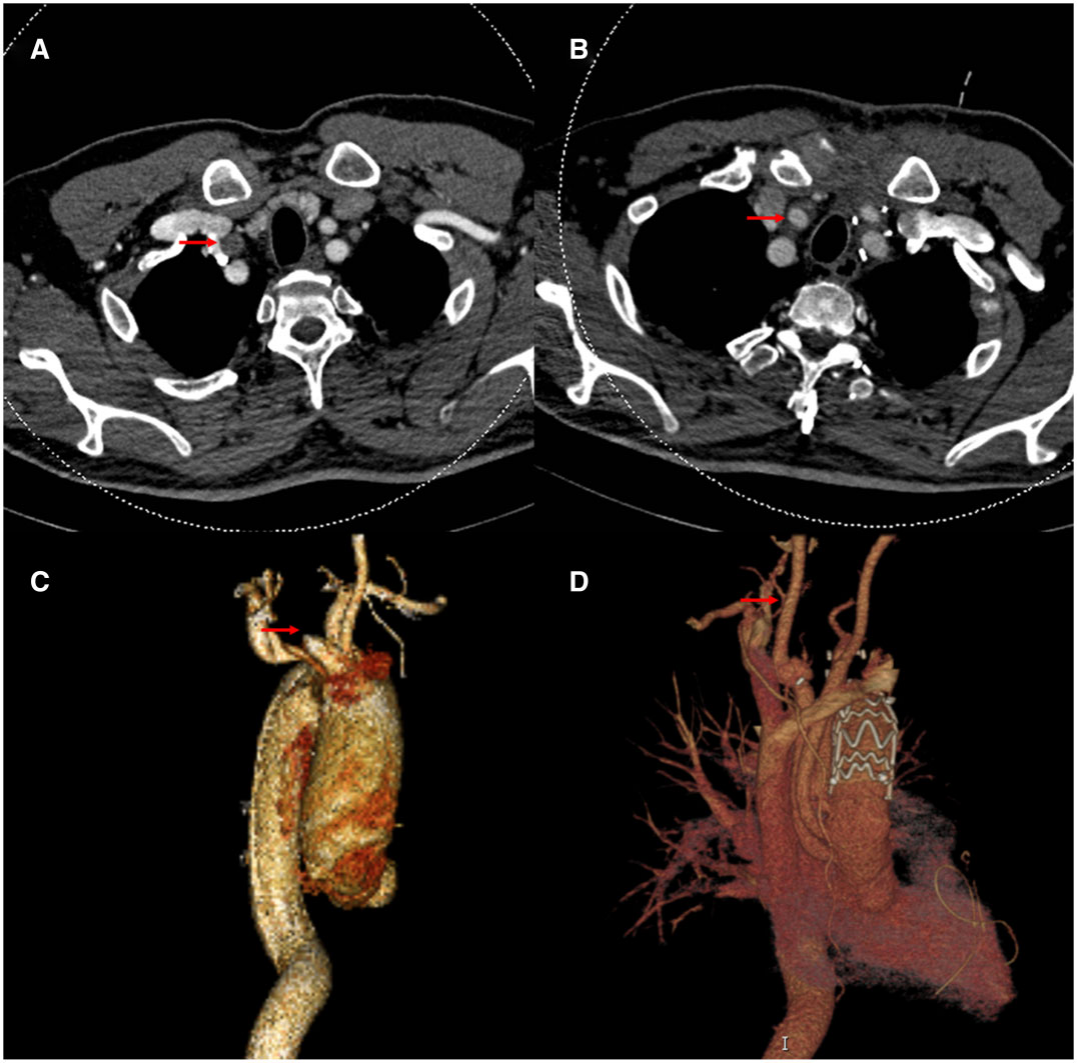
Computed tomography angiography (CTA) shows acute type A aortic dissection complicated with right carotid artery occlusion (A and C). CTA shows patent right carotid artery, successful replacement of ascending aorta and implantation of thoracic stent after discharging 3 months later (B and D).
